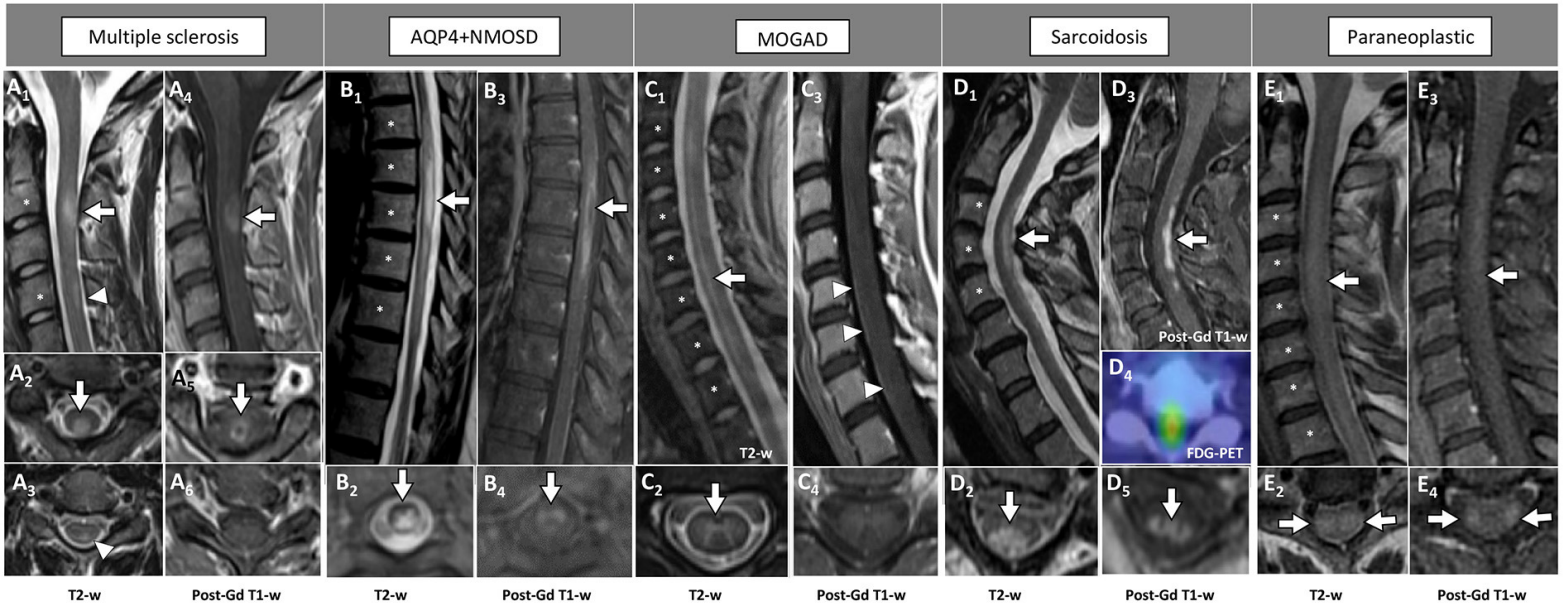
Examples of inflammatory myelopathies on MRI. The extension of the T2-hyperintensities along consecutive vertebral segments is highlighted by asterisks. [(A) MS] Acute short cervical lesion with focal spinal cord swelling [(A_1_) arrow] involving the central gray matter and the dorsal columns [(A_2_) arrow], showing homogeneous enhancement on sagittal view [(A_4_) arrow] and a circular ring of enhancement on axial view [(A_5_) arrow]. A chronic lesion at the level of C4 is also observed [(A_1_) arrowhead], located in the dorsal columns [(A_3_) arrowhead] and not showing contrast enhancement (A_6_). [(B) AQP4+NMOSD] Thoracic spinal cord lesion, longitudinally extensive on T2-weighted images [(B_1_) arrow], centrally located [(B_2_) arrow], showing lens-shaped ring enhancement on sagittal view [(B_3_) arrow], and inhomogeneous enhancement on axial view [(B_4_) arrow]. [(C) MOGAD] Cervico-thoracic spinal cord lesion, longitudinally extensive on T2-weighted images characterized by a “sagittal linear” aspect [(C_1_) arrow] corresponding to a selective involvement of the gray matter (“H-sign”), on axial view [(C_2_) arrow]. Parenchymal enhancement is absent (C_3_,C_4_), although a subtle leptomeningeal enhancement is detected [(C_3_) arrowheads]. [(D) Sarcoidosis] Cervical spinal cord lesion, longitudinally extensive on T2-weighted images [(D_1_) arrow] involving the central gray matter and the dorsal columns [(D_2_) arrow], with dorsal enhancement on sagittal view [(D_3_) arrow] and “trident sign” on axial view [(D_5_) arrow]. Increased glucose uptake is also observed, corresponding to an area of red color in the positron-emission tomography (D_4_). [(E) Paraneoplastic] Cervical spinal cord lesion, longitudinally extensive on T2-weighted images [(E_1_) arrow] involving the lateral white matter tracts bilaterally [(E_2_) arrows], showing subtle enhancement on sagittal view [(E_3_) arrow] and axial view [(E_4_) arrows]. FDG-PET, ^18^F-Fluorodeoxyglucose-positron emission tomography; Gd, gadolinium; T2-w, T2-weighted; T1-w, T1-weighted.
